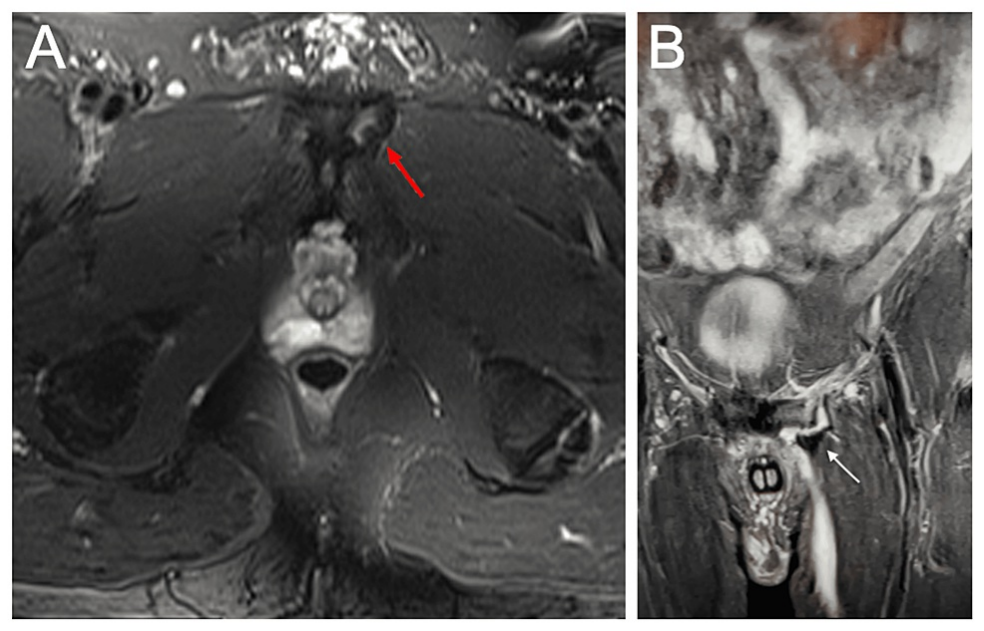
Axial (A) and coronal (B) T2 fat saturation MRI images through the symphysis pubis. A: T2 axial imaging displays an amplified signal within and around the anterosuperior segment of the pubic body (red arrow). This signal is discernible directly under the insertion point of the common adductor-rectus abdominis aponeurosis, indicating long-term tendon and bone interface disruption. B: Tear of the adductor longus muscle tendon (white arrow) from the symphysis with slight retraction and fluid (white signal) between the tendon and bone.
